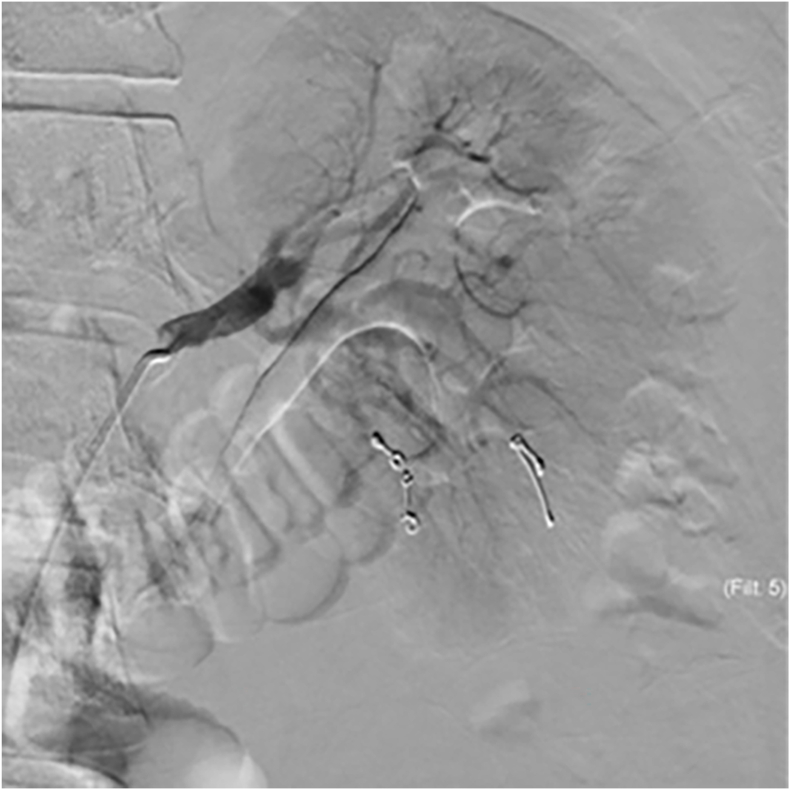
Coils were deployed into interlobular arteries based on the site of laceration and location of extravasation on the previously obtained CT images. DSA post coiling was performed.
